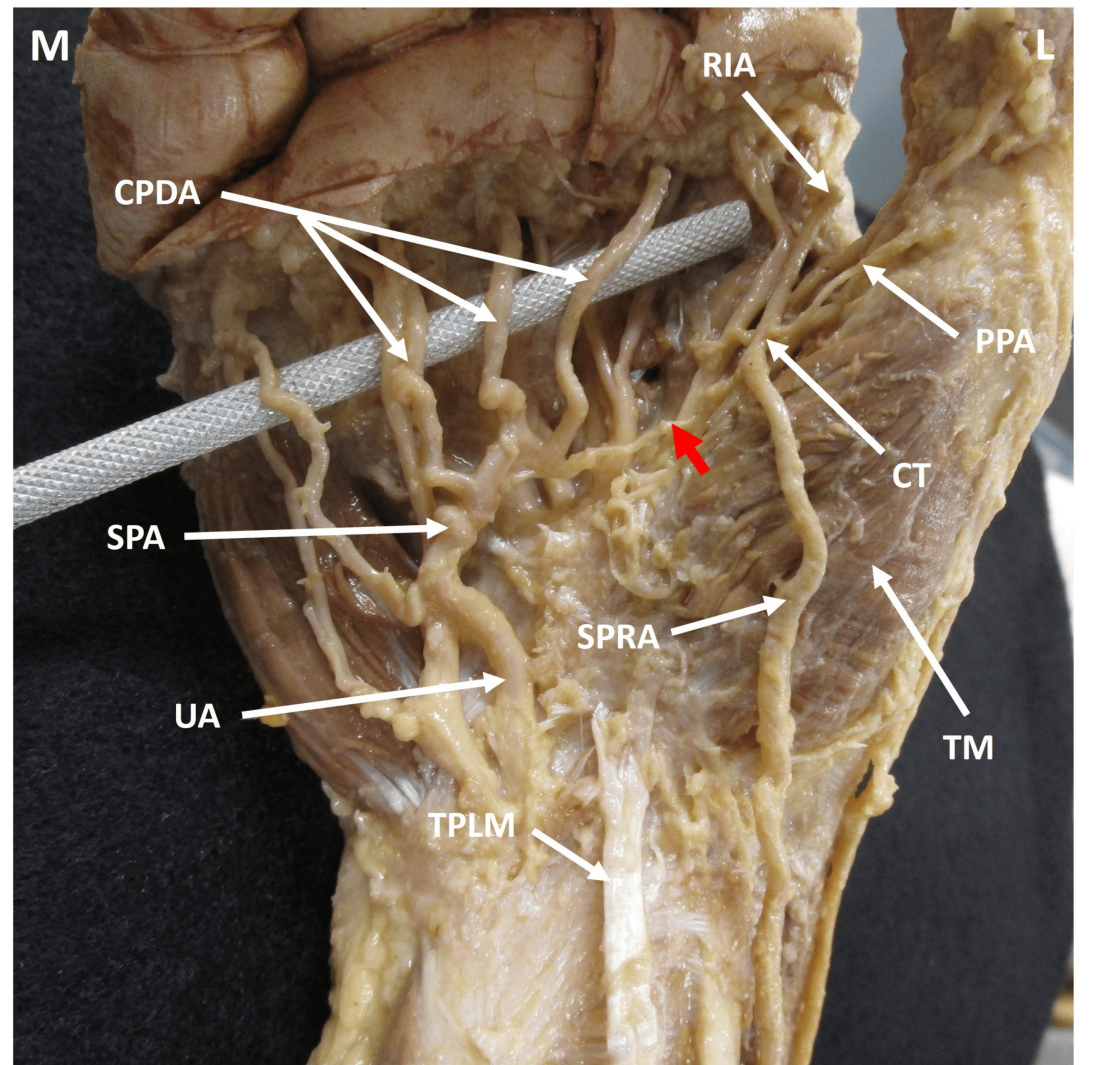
Anterior view of the right hand demonstrating the vasculature. Superficial palmar branch of the radial artery coursed superficial to the thenar muscles before providing a communicating branch (red arrow) to the superficial palmar arch. The superficial palmar branch of the radial artery provided a common trunk which gave rise to both the princeps pollicis and radialis indicis arteries. The 2nd – 4th common palmar digital arteries branched from the superficial palmar arch. CT: common trunk; SPRA: superficial palmar branch of radial artery; RIA: radialis indicis artery; PPA: princeps pollicis artery; CPDA: common palmar digital arteries; UA: ulnar artery; TM: thenar muscles; TPLM: tendon of palmaris longus muscle; SPA: superficial palmar arch; M: medial; L: lateral.
